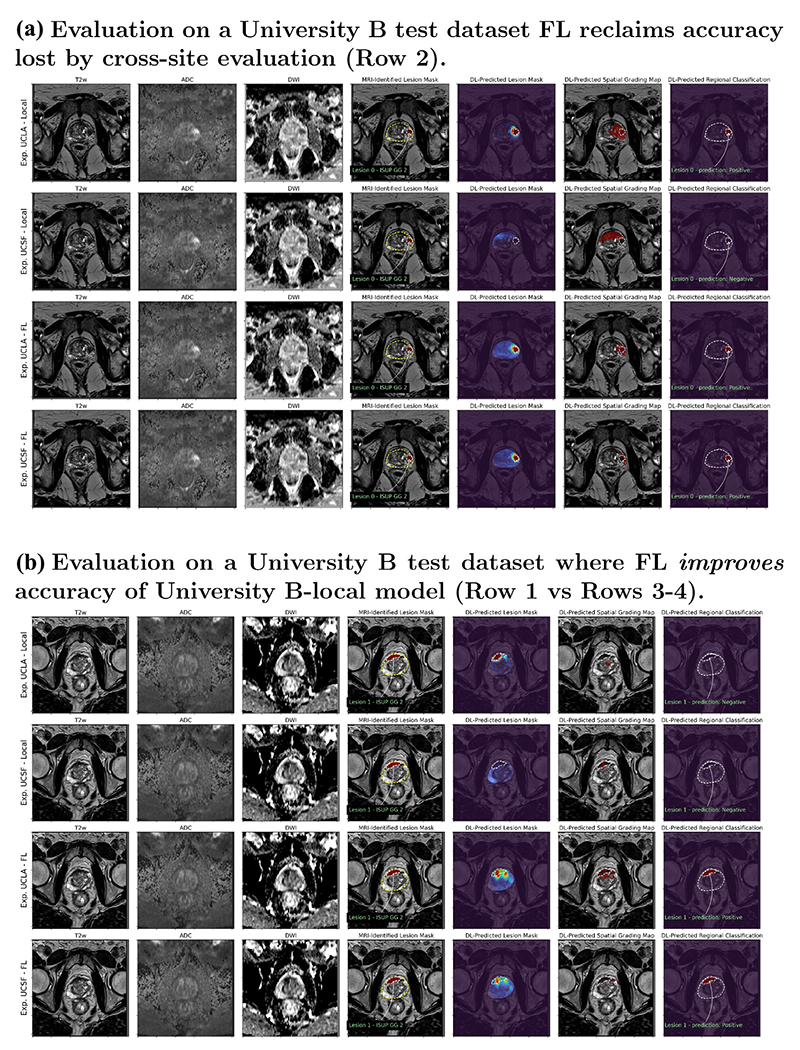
Evaluation of UCNet models on example UCLA datasets. (A) depicts a transverse slice of an exam with a MRI-identified ISUP GG 2 lesion where UCSF-local model performs poorly, but both federated models (Rows 3-4) achieves the same level of accuracy as UCLA-local model. (B) depicts a transverse slice of an exam with a MRI-identified ISUP GG 2 lesion where both local models performs poorly, but federated models perform well.
